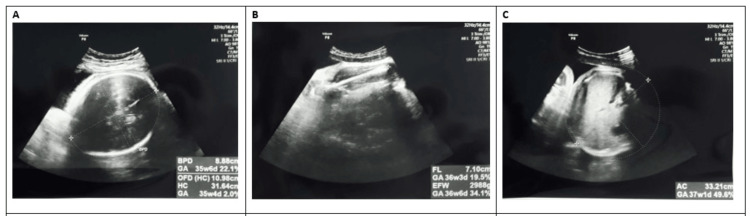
Ultrasound measurement of standard fetal biometry:(A) biparietal diameter and head circumference, (B) femur length, and (C) abdominal circumference.
